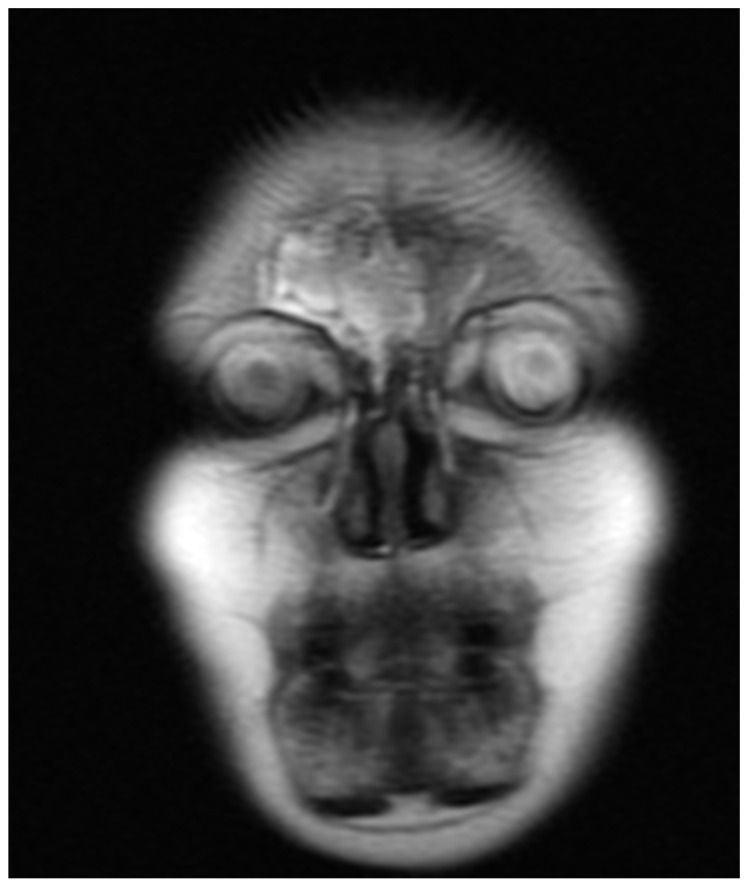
MRI of the head and axial plane revealed frontal sinus involvement, more severe on the right side.
